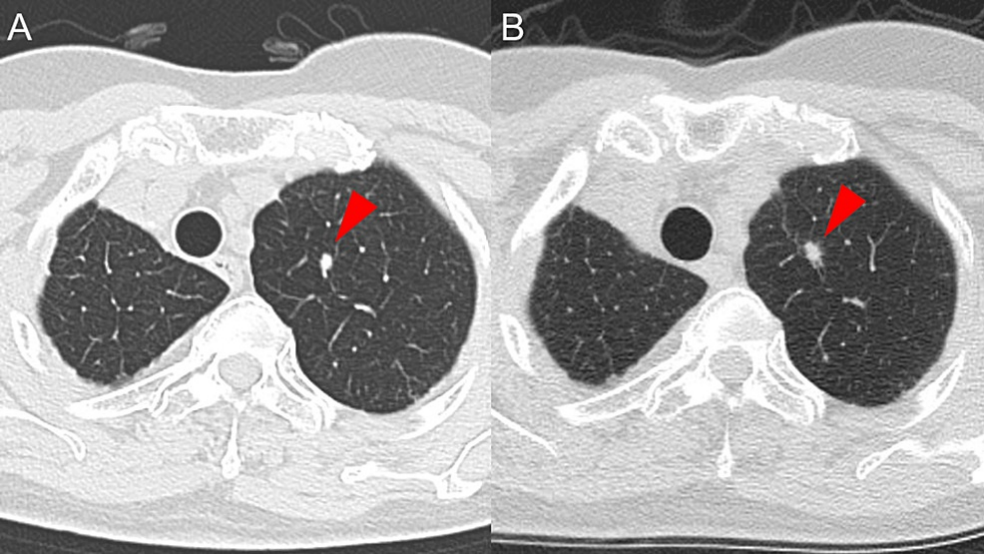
A series of chest computed tomography during pembrolizumab treatment; at the initiation (A) and nine months after the initiation of the treatment (B), showing stable disease.
